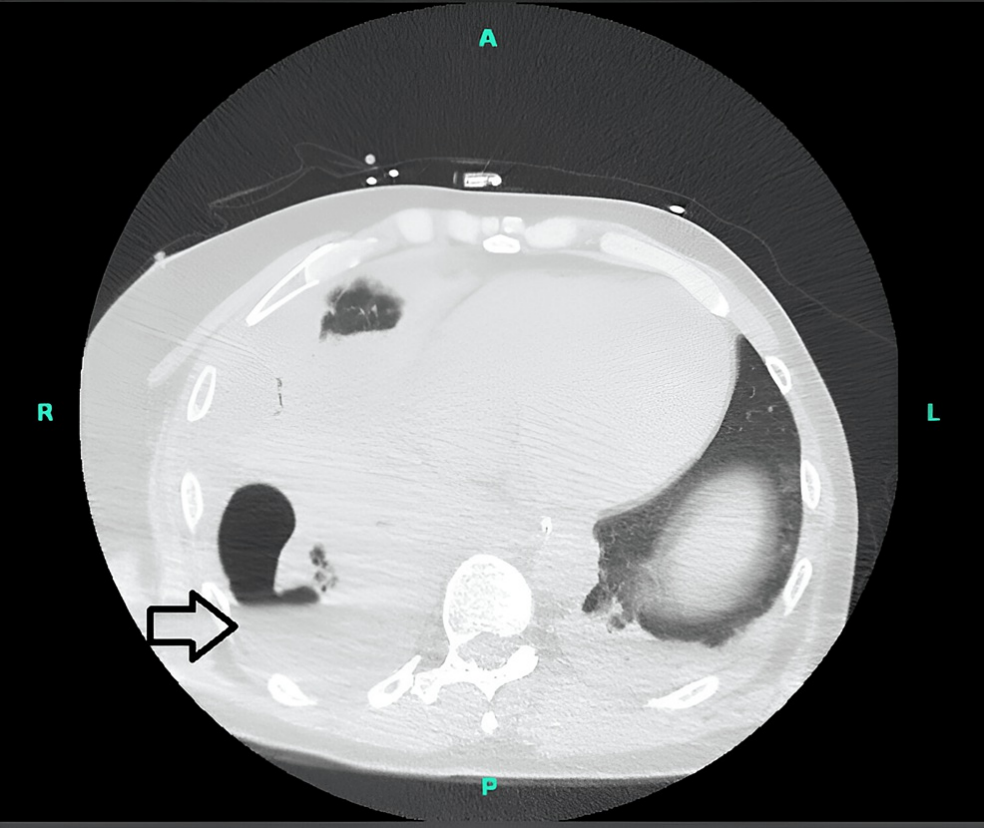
CT chest on day 4 of hospital admission revealed right-sided empyema thoracis (black arrow)
